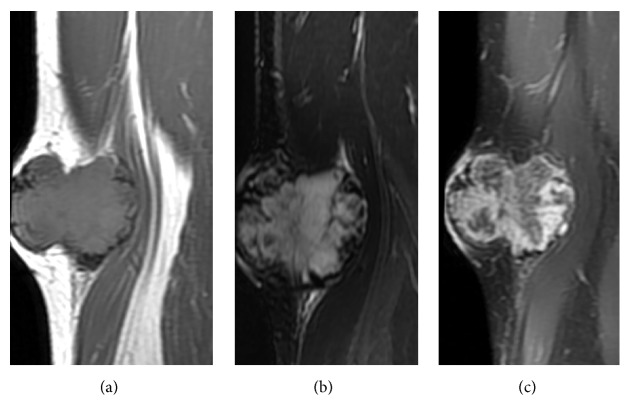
Typical radiological appearance from a 40-year-old man with EMC of the right thigh. An irregular-shaped soft tissue mass in right thigh with long T1 and T2 was observed (a, b). An enhanced MRI scan revealed an obvious uneven enhanced mass with radiated arrangement separation enhanced like spokes (c).
